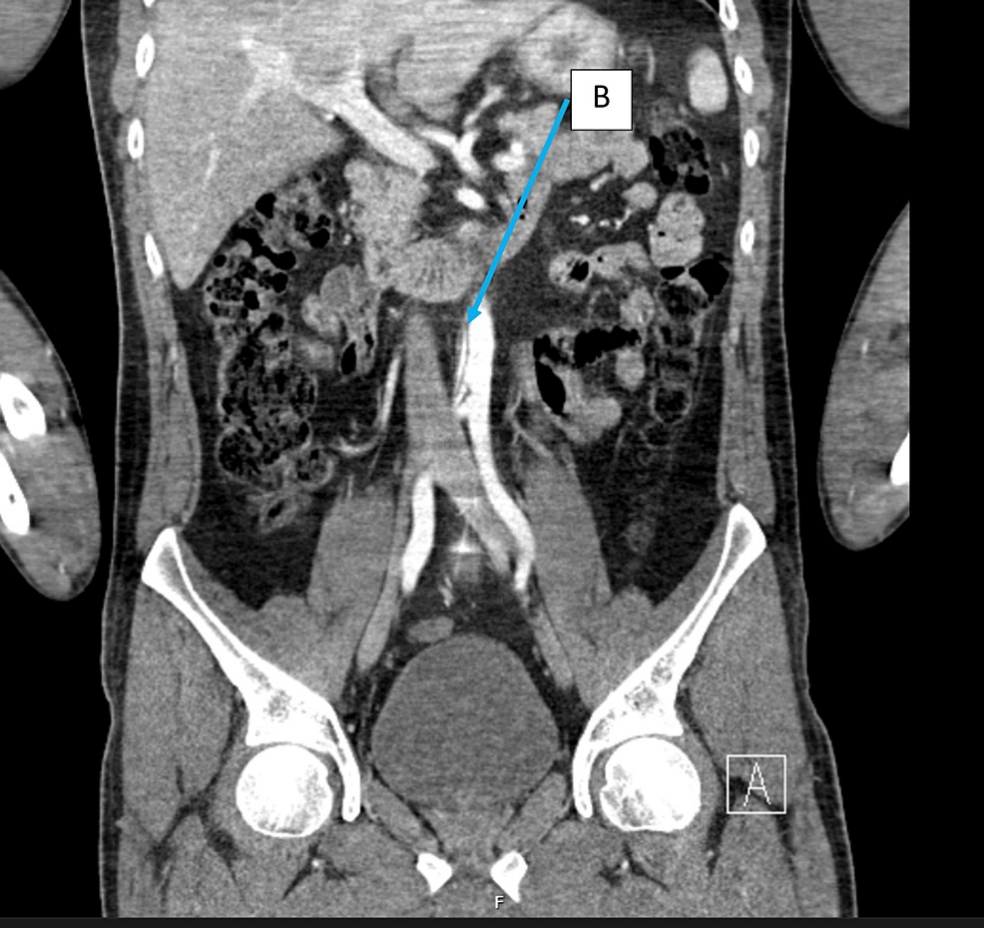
Coronal section computed tomography (CT) imaging with contrast showing an intimal tear and aortic dissection (B) in the infrarenal abdominal aorta.
